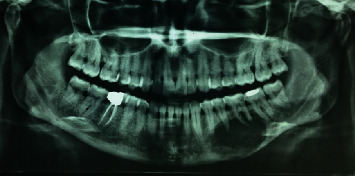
Panoramic radiograph showing a radiolucent lesion in the left mandible body containing small radiopaque foci, especially in the lower parts.
